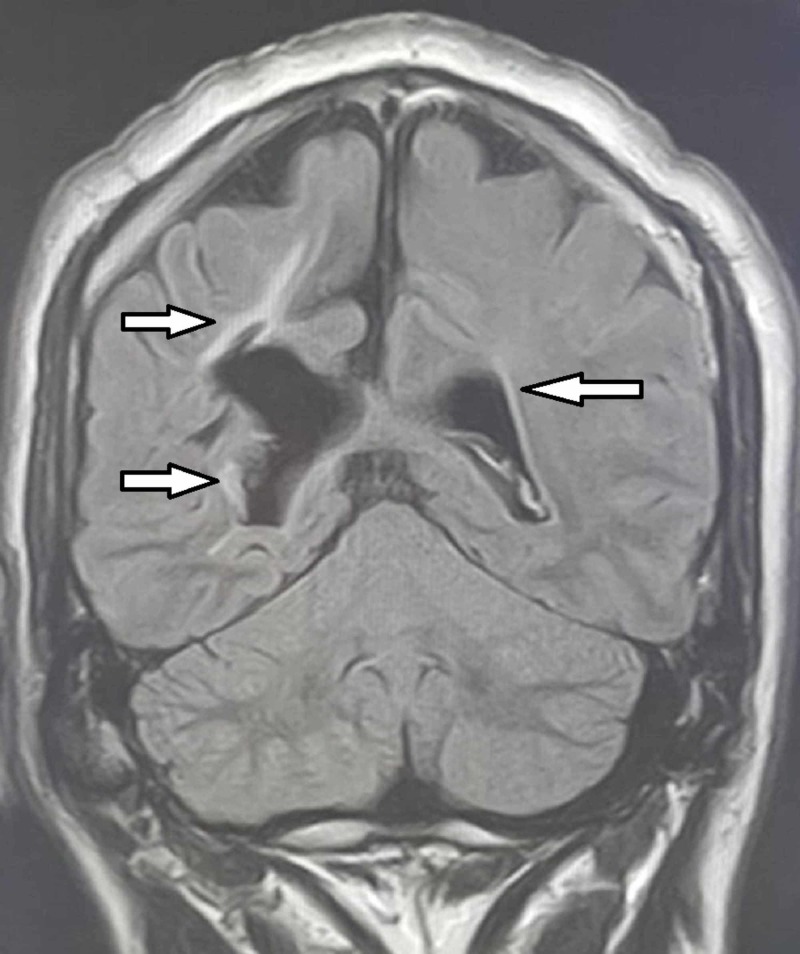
MRI sagittal section showing periventricular hyperintensities (Arrows)
